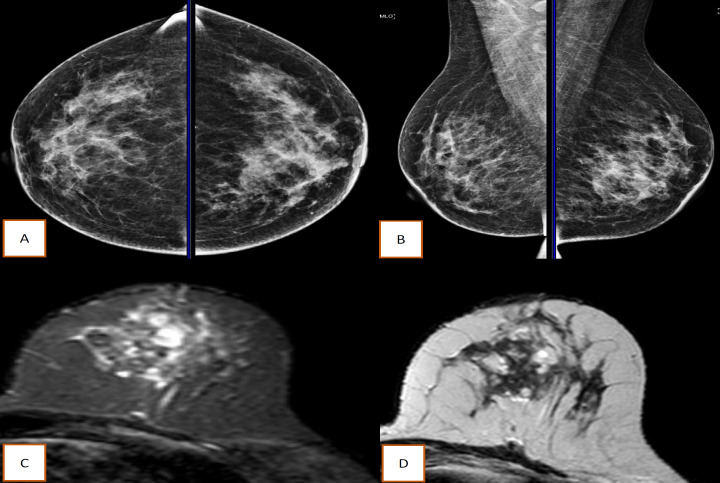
Mammographic and MRI features of MBC. A & B, Focal asymmetry in the left breast without any lump on mammography; C, MRI of the left breast showing T2 hyperintensity; D, Same lesion showing peripheral enhancement with surrounding non-mass clustered rim enhancement on the diffusion weighted imaging sequence of MRI
